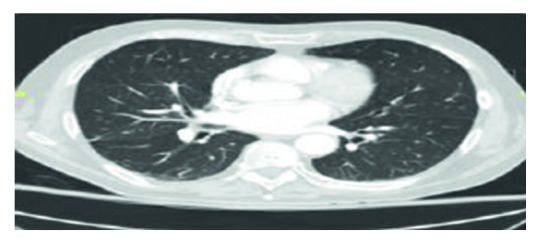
Chest CT scan 3 months posttreatment showing interval improvement of bilateral opacities.
